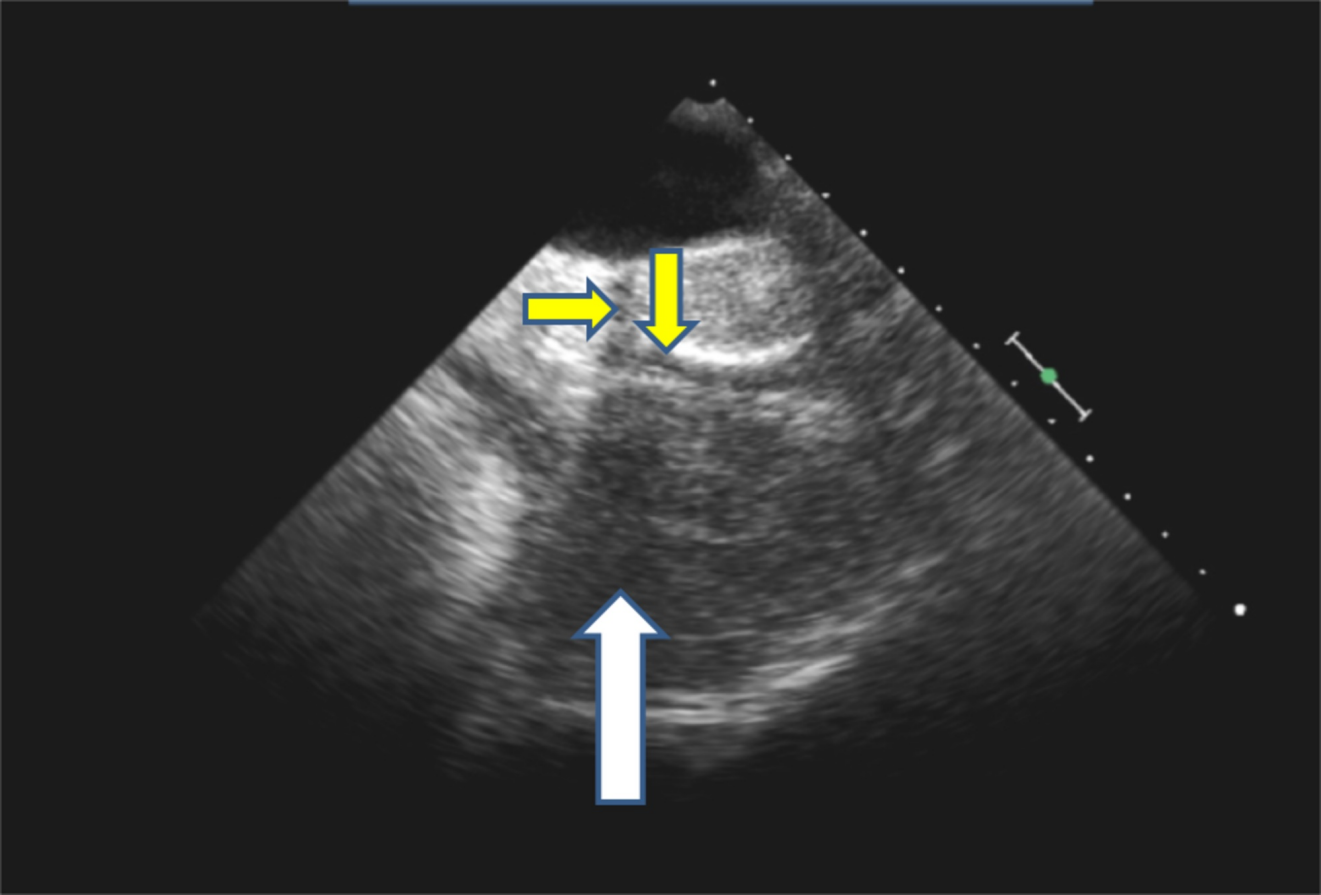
Pre-surgery transesophageal echocardiogram (TEE) image without color flow showing the pericardial hematoma Pre-surgery TEE showing pericardial hematoma (white arrow) compressing and nearly obliterating the right atrium (slit-like shape, yellow arrows). Image seen is without color flow.
